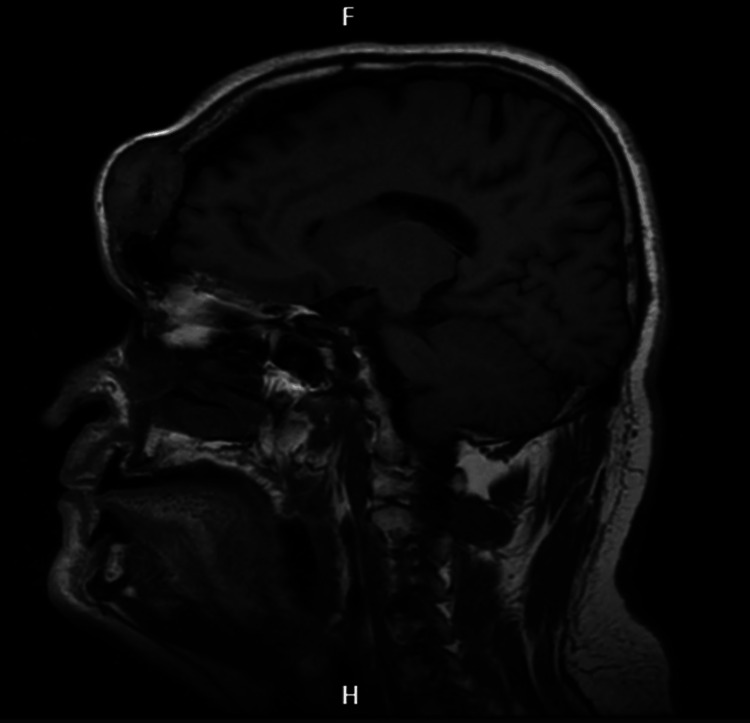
Cranioencephalic MRI in sagittal section The image shows a sagittal section in T1 fluid-attenuated inversion recovery (FLAIR).
